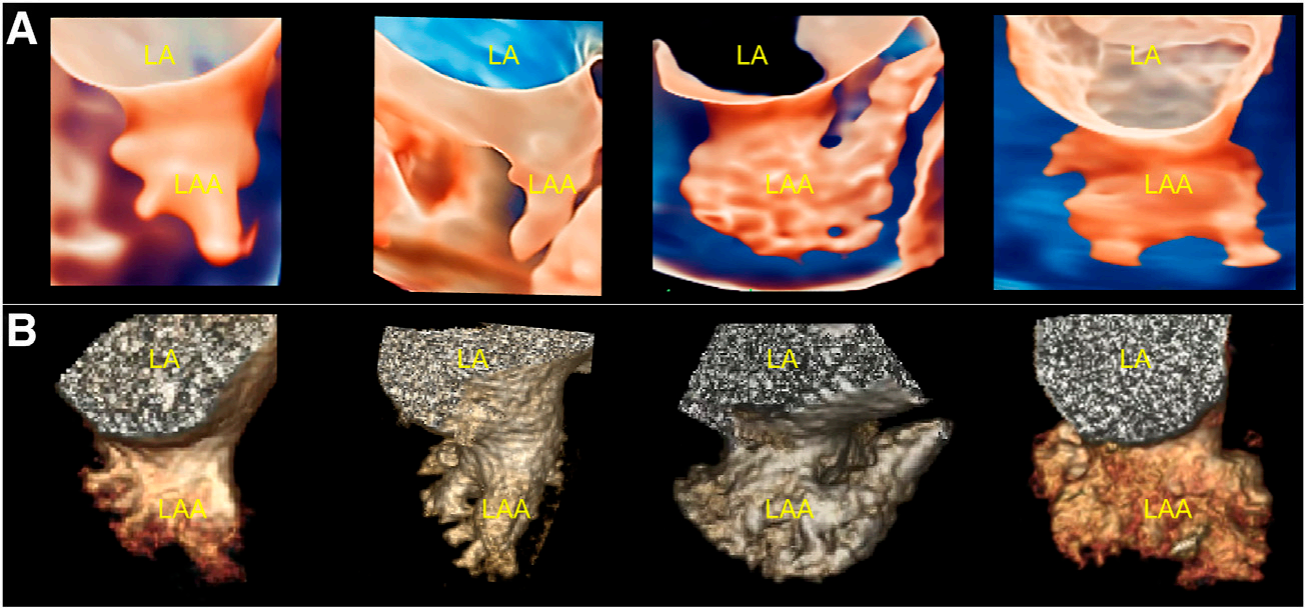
Comparison of TrueVue Glass and CCTA for displaying different morphologies of LAA. (A). From left to right, TrueVue Glass shows cactus, windsock, chicken-wing, and cauliflower type of LAA (B). From left to right, CCTA display of the above morphological types of LAA are shown. CCTA, cardiac computed tomographic angiography; LA, left atrium; LAA, left atrium appendage.
